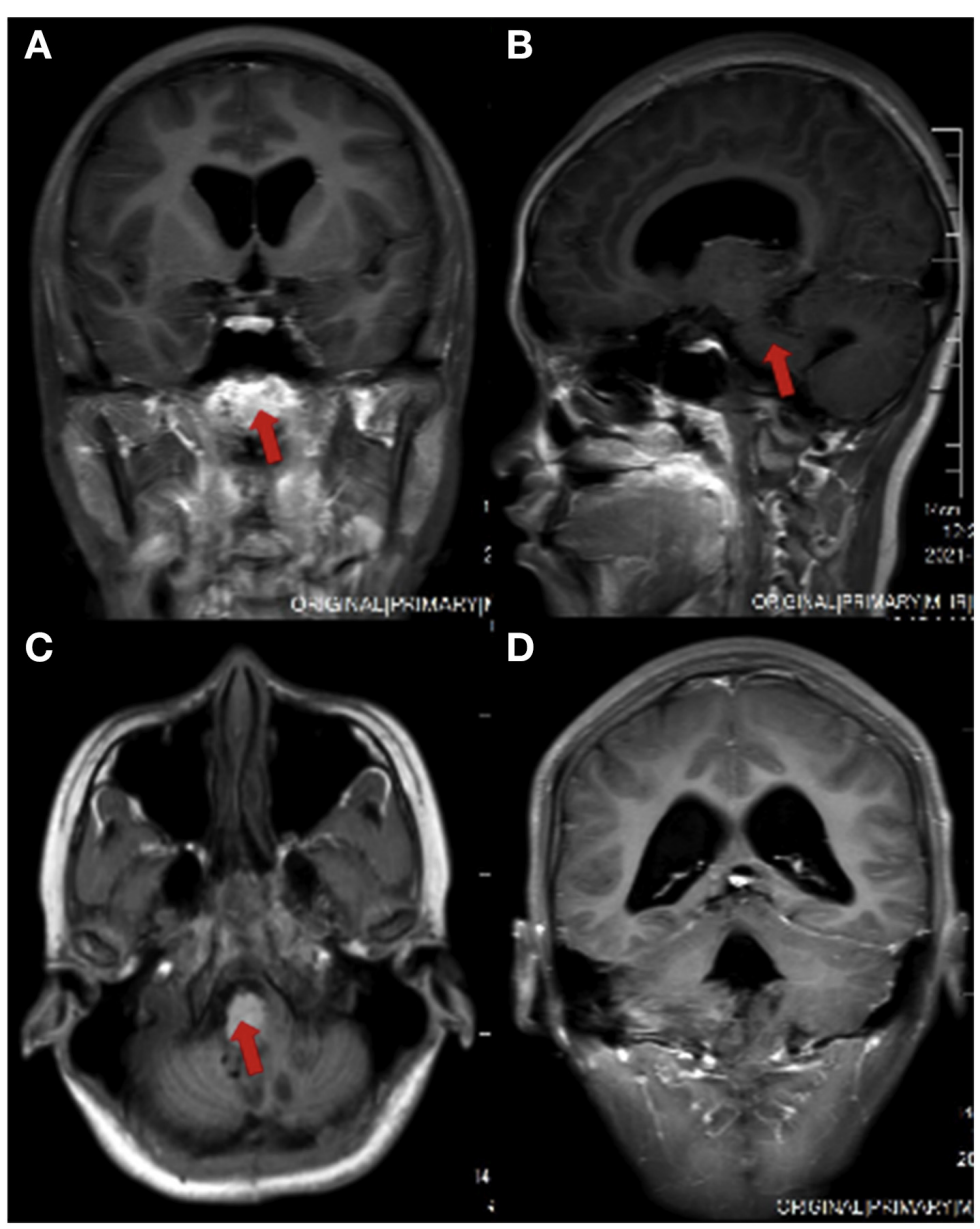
Head MRI of the patient before surgery. (A) coronal view; (B) sagittal section view; (C) axial view; (D) ventricular enlargement in coronal view
